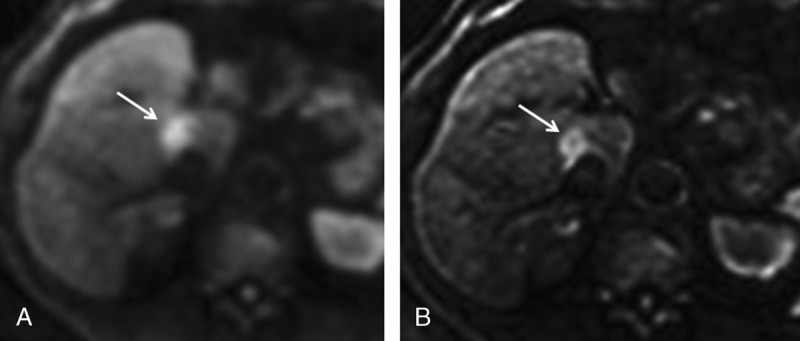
HCC in a 69-year-old man. A, cc-DWI; B, hc-DWI. The HCC (arrow) is more clearly visualized on the hc- than the cc-DWI.
